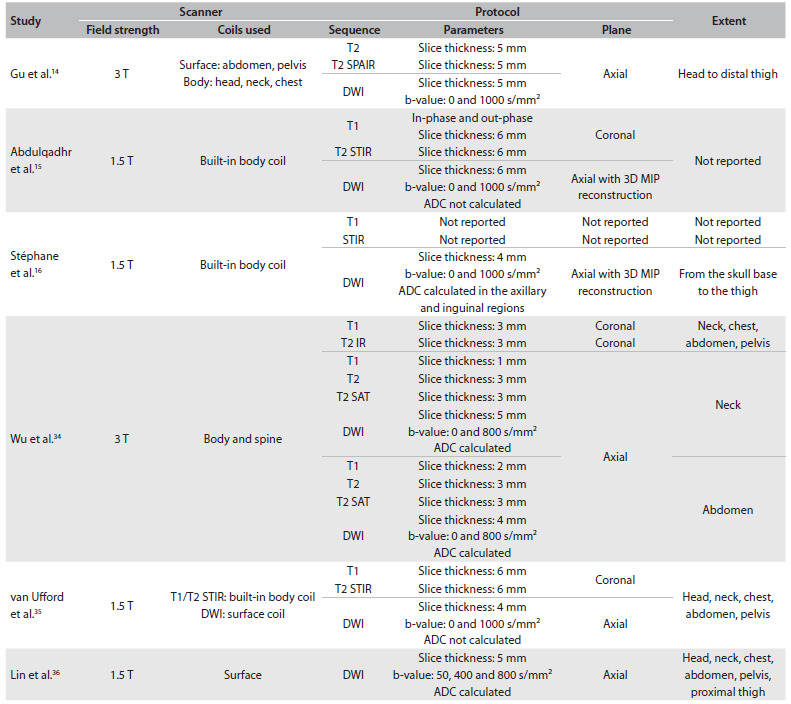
WB-MRI protocols used in studies included in the meta-analysis
